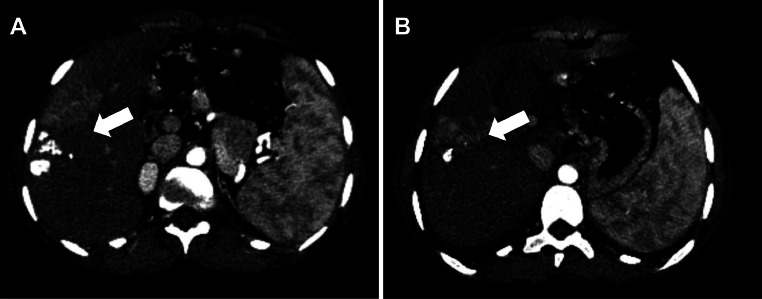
Axial CT scan showing a complete Lipiodol uptake in embolized areas; absence of new enhancing lesions; stasis achieved in targeted artery (white arrow).
